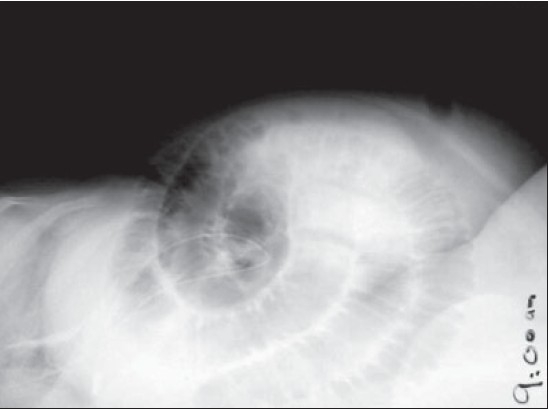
Plain X-ray abdomen showing the air filled loop of small bowel in the umbilical port site
